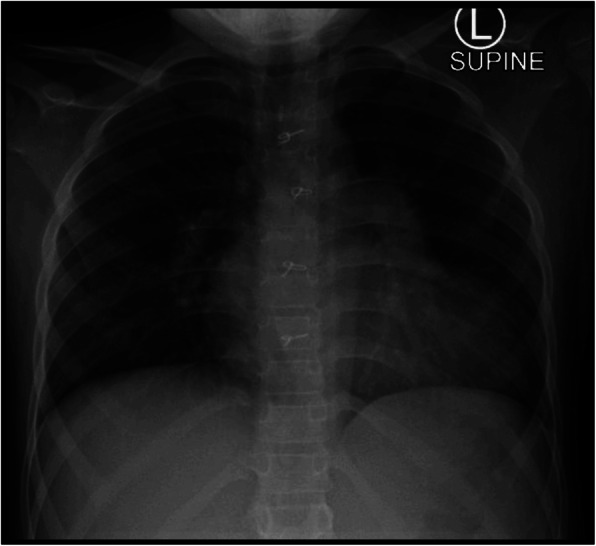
Chest X-ray showing that the cardiac silhouette is enlarged with the prominence of the left pulmonary artery. The lung fields and the costophrenic angles are clear
